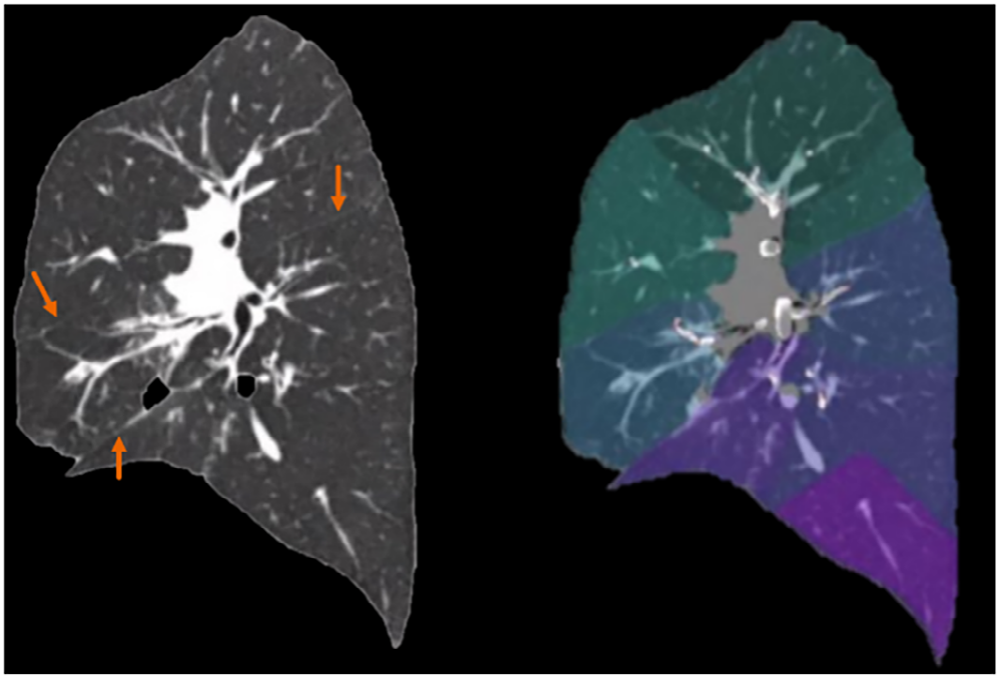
Lung Parcellation using Deep Image‐to‐Image Network. A sample case showing the challenging task of lung segment parcellation directly from CT data. Fissures are noted with orange arrows. There are no clear visible boundaries between most adjacent segments, and meticulous navigation on the CT is required while evaluating bronchi and pulmonary vessel pathways. Left: CT slice with visible lung fissures shown in orange arrows. Right: automatic segment parcellation results on the same CT slice. CT, computed tomography
